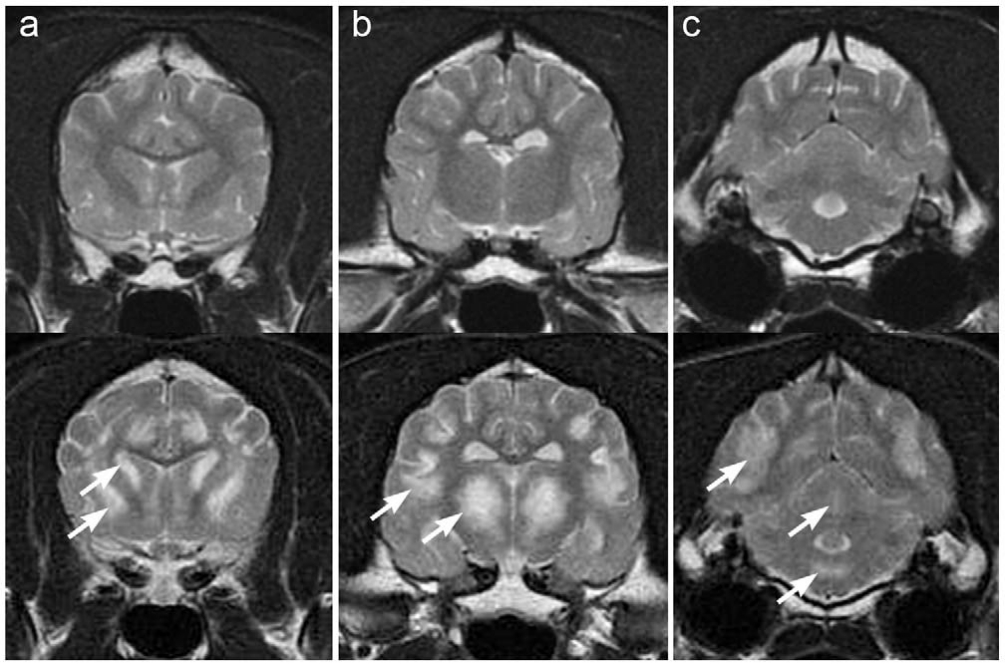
(upper panel). MRI images (T2-weighted, transverse) of a normal brain from a clinically normal 1 year old male Alaskan Husky (C#1). Figure 1
